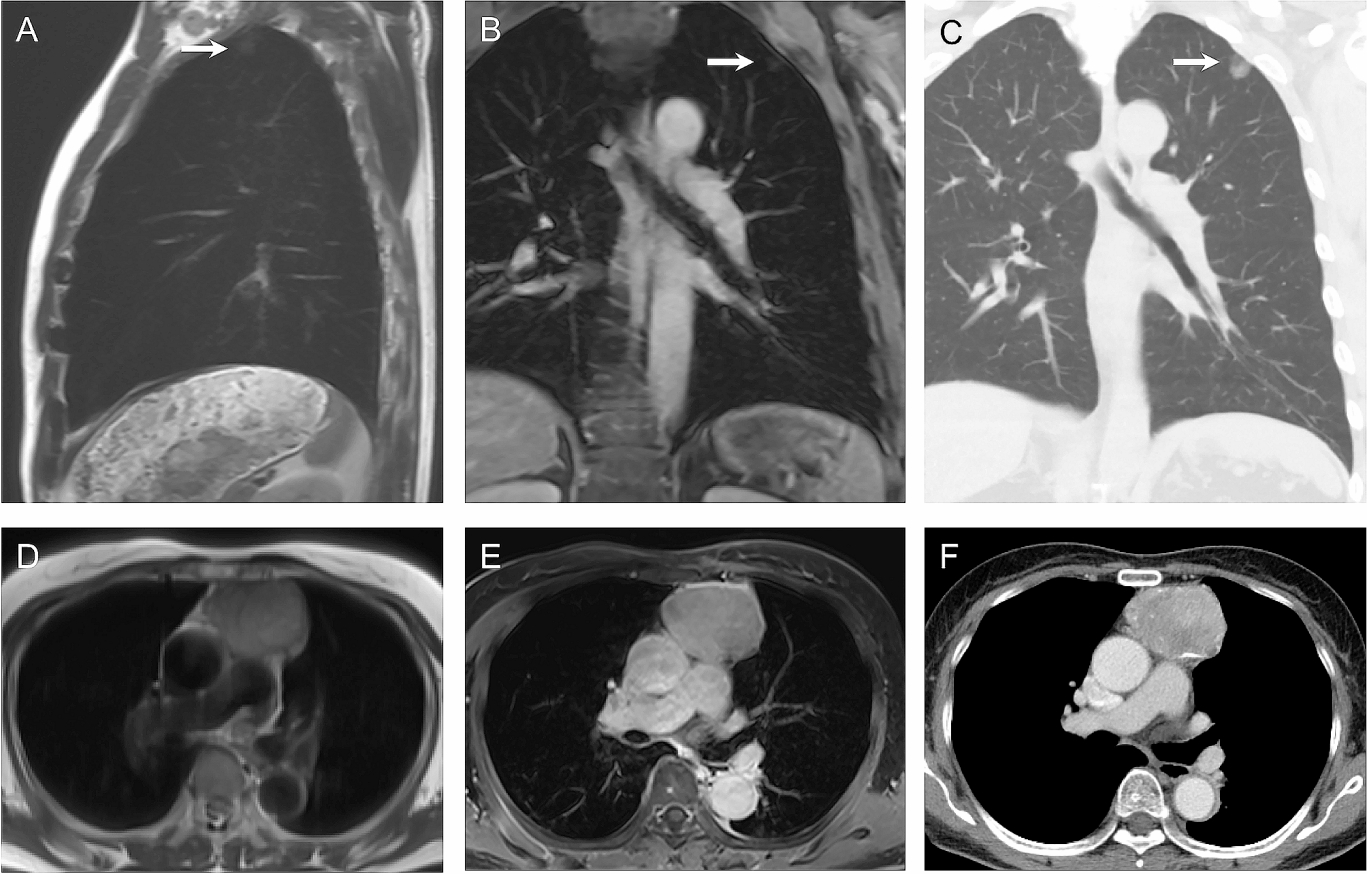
Imaging findings with Oncologically Relevant Findings Reporting and Data System category 4 in chest region. Sagittal T2 half-Fourier single-shot turbo spin echo (HASTE) (A) and coronal contrast-enhanced T1 gradient echo (GRE) (B) images reveal 17-mm subpleural nodule (arrows) in upper lobe of left lung in 62-year-old man. Lesion is seen on corresponding coronal CT scan lung window (C) and was confirmed as lung adenocarcinoma after surgical resection. In 69-year-old woman, 5.8-cm anterior mediastinal mass with hyperintensity was noted on T2 HASTE image (D) and contrast enhancement on T1 GRE image (E). Corresponding axial CT scan (F) demonstrates calcification in mass and thymoma was diagnosed after surgical resection
